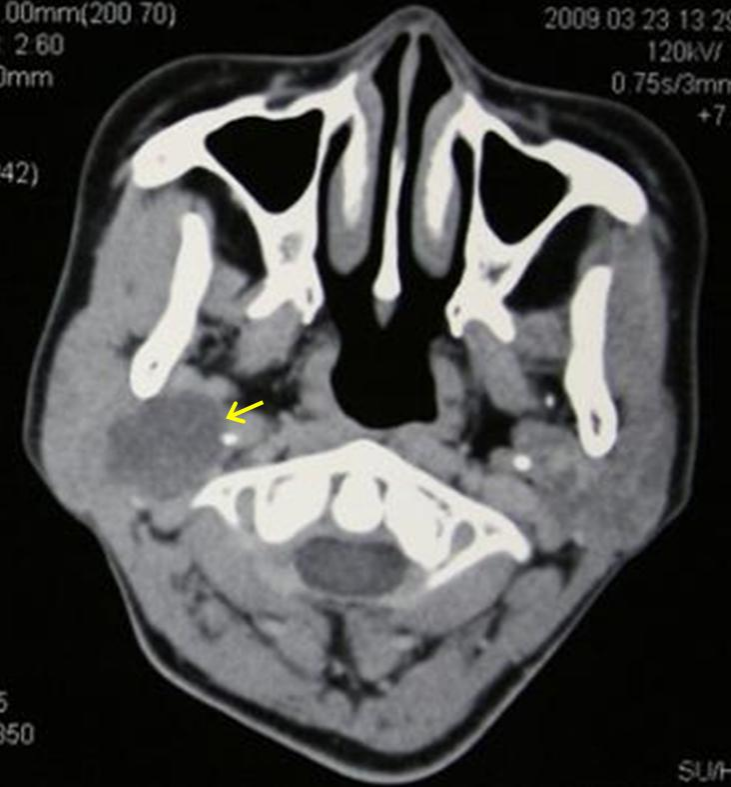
CT scan of the patient. A contrast enhanced Computed tomography (CT) scan revealed a well defined soft tissue mass (40 mm × 25 mm, arrow) in the right deep parotid lobe.
